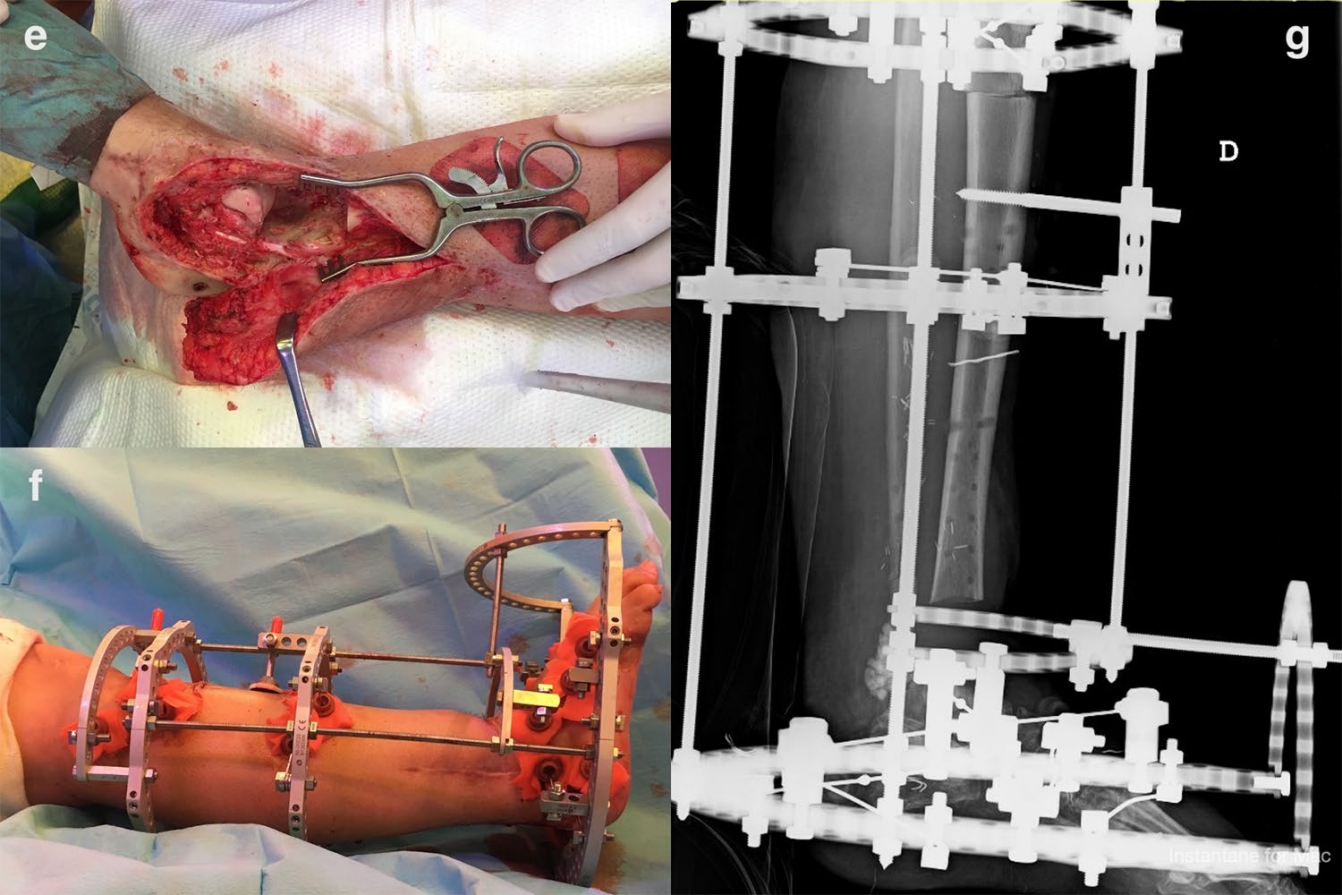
Panel of images demonstrating the 3-Stage Protocol; e third-stage procedure, previous (second stage) soft-tissue coverage obtained through a free anterolateral thigh (ALT) flap to reconstruct the massive soft-tissue defect; during the third stage, the flap is lifted and the spacer is extracted (notice the dome of the talus); f definitive reconstruction of the segmental bone defect is achieved using a tibiotalar arthrodesis through circular-frame bone transport; g postoperative radiograph after the third stage has been completed
